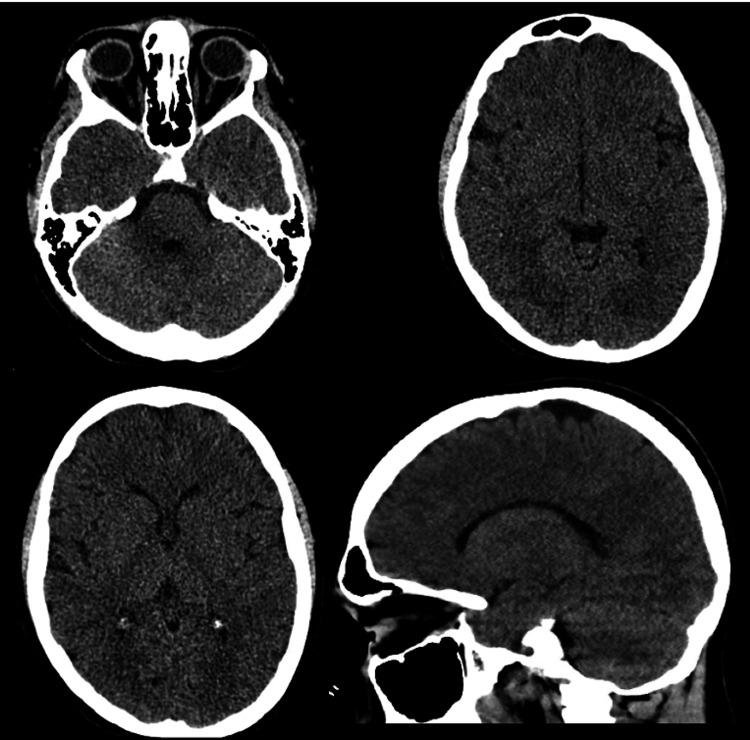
Head CT Noncontrast head CT obtained in the emergency department reported as sinusitis without acute intracranial findings.
